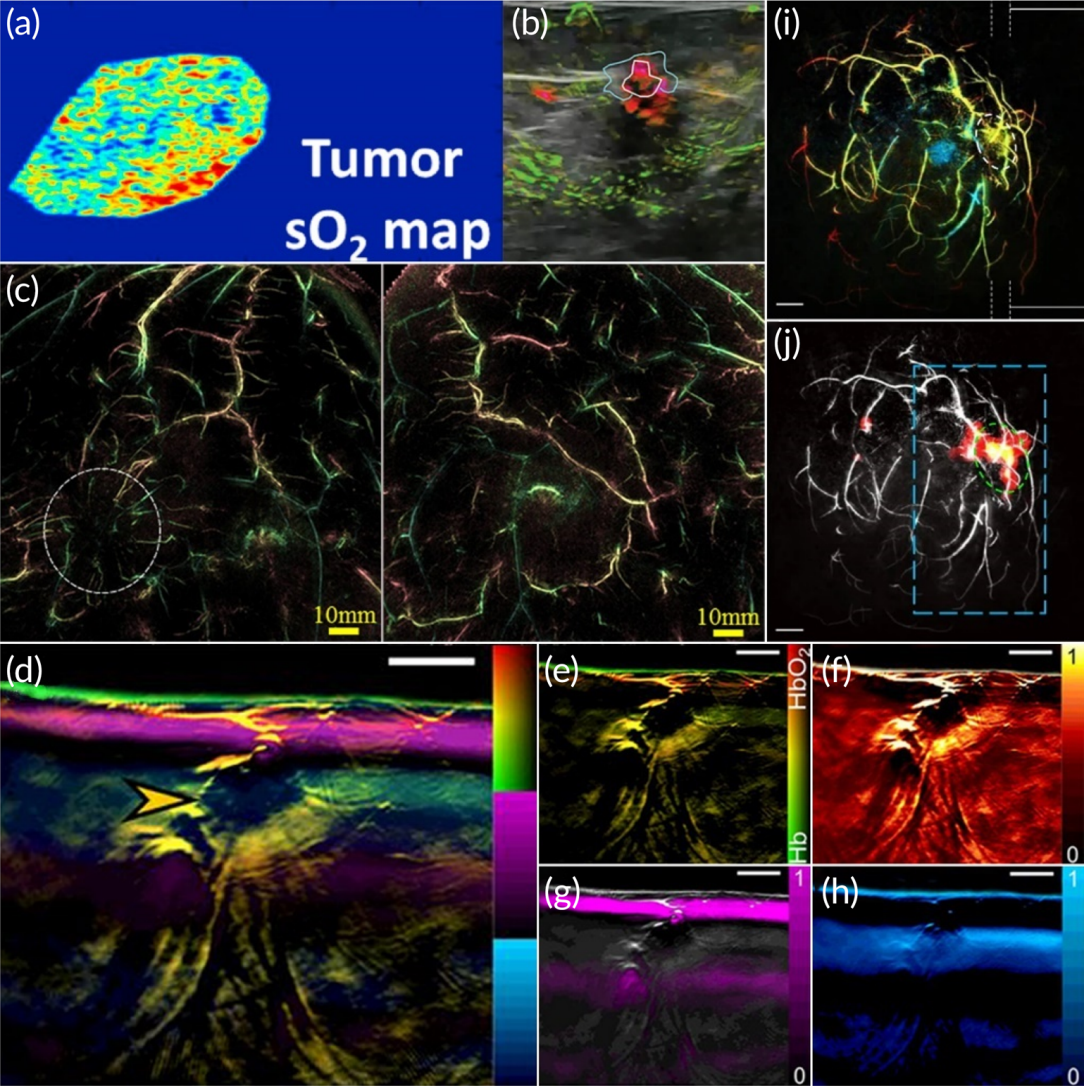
Photoacoustic computed tomography (PACT) imaging of breast cancer. (a) Calculated sO_2_ map of the breast tumor via PACT.^149^ (b) Ultrasound and PACT combined images of invasive mixed ductal and lobular carcinoma in the breast, with oxygenated blood as green and relatively deoxygenated blood as red.^150^ (c) PACT images (maximum intensity projection) of the breast tumor (left) and the contralateral side (right). The location of the tumor was indicated by the dashed circle. Specific structure of blood vessels could be seen around the tumor, whereas not in the contralateral side.^151^ (d) Unmixing multispectral optoacoustic tomography (MSOT) image of the breast tumor showing the four key absorbers.^133^ (e) MSOT image of hemoglobin and oxyhemoglobin. (f) MSOT image of total blood volume. (g) MSOT image of lipid. (h) MSOT image of water content. (i) Single breath‐hold (SBH)‐PACT angiograms of invasive ductal carcinoma in the breast.^22^ (j) Automatic tumor detection on vessel density maps by SBH‐PACT.^22^
